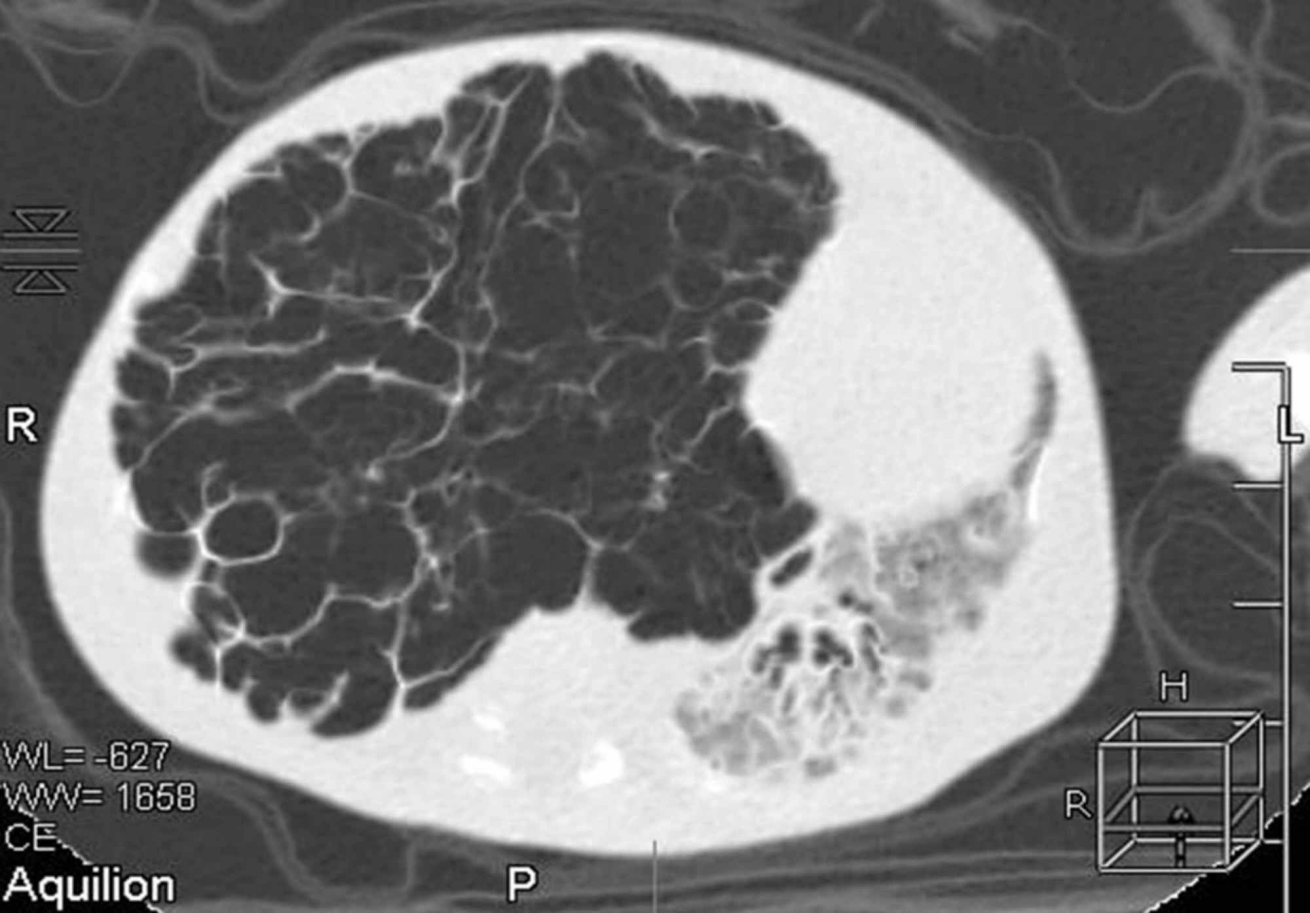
Chest computed tomography (CT), axial view, showing hyperinflation of the right middle lobe, mediastinal shift to the left, and remaining atelectatic tissue
